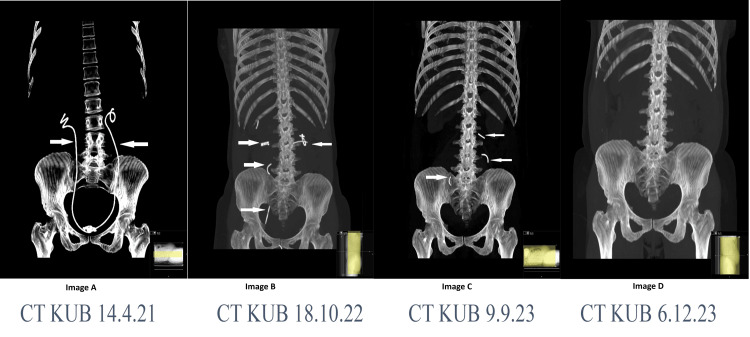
MIP (maximum intensity projection image) images from coronal sections of a CT kidney, ureter and bladder with a modified bone window showing gradual spontaneous fragmentation and disappearance of bilateral double J stents over time. Figure 2. Serial maximum intensity projection (MIP) CT images of the kidneys, ureters, and bladder (KUB) demonstrating gradual spontaneous fragmentation and disappearance of bilateral double J stents over time. (A) April 14, 2021: Intact bilateral ureteral stents in situ (white arrows) seen extending from the kidneys to the urinary bladder. (B) October 18, 2022: Multiple fragmented stent pieces along the ureters (white arrows) are seen within the kidneys and the right ureter, with the disappearance of most of the stent fragments from the left ureter and the bladder. (C) September 9, 2023: Further fragmentation with only small fragments remaining (white arrows) within the left kidney and proximal right and left ureters. (D) December 6, 2023: Complete disappearance of both stents
